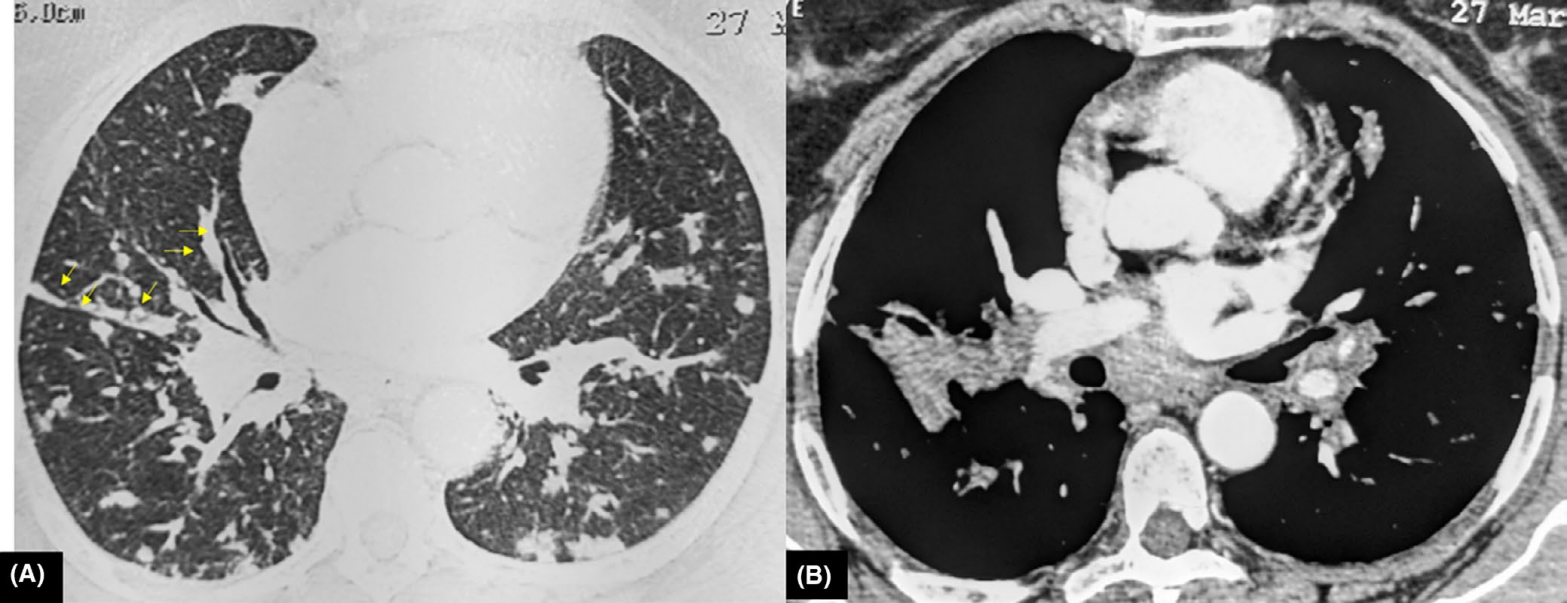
Axial CT of the chest (A, B) shows small nodules in a perilymphatic distribution along interlobular septa and the peribronchovascular (arrows)
